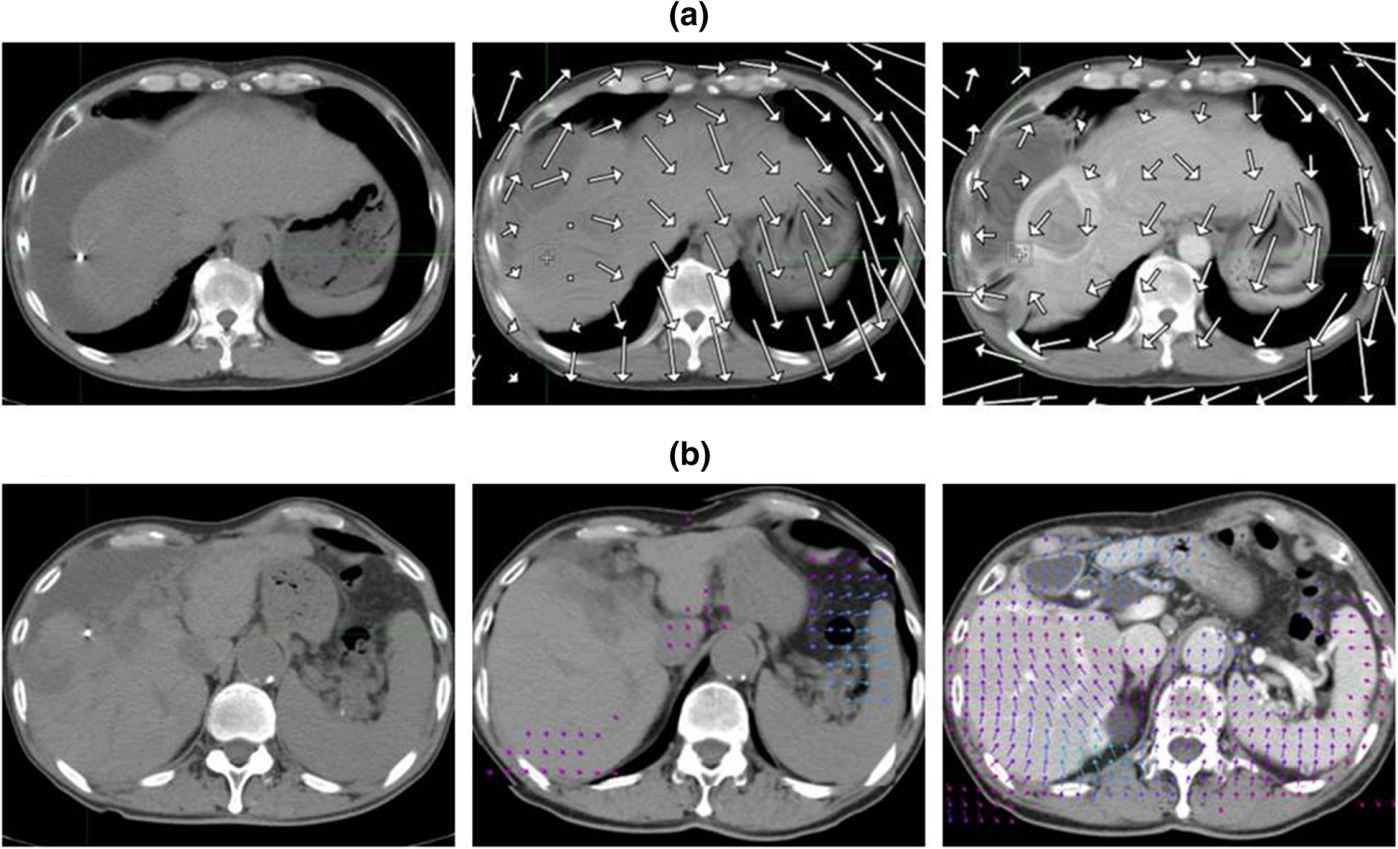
Cases with large discrepancies in the deformation pattern between plain and contrast-enhanced CT. a Proton beams at doses of 50 GyE were delivered to the tumor in S8 11.2 months before. Left: posttreatment CT; middle: deformed plain CT; right: deformed contrast-enhanced CT. The vector expressed by MIM means the fiducial registration error in each area. b Proton beams at doses of 70 and 66 GyE were delivered to the tumor in S6 and S8 12.7 months before. The vector expressed by Velocity means the fiducial registration error in each area
